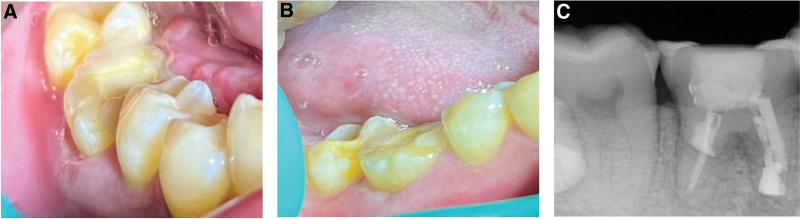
Clinical and radiographic examination with favorable prognosis at 3-month follow-up.
